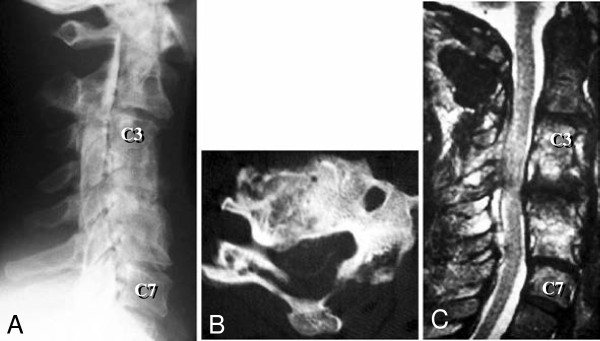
Three images obtained upon admission to our hospital (4.5 months after the patient’s third surgery). (A) A lateral view myelogram showing anterior compression of the dural tube at the C4-C5 level. (B) An axial computed tomographic myelogram showing that the spinal cord is compressed from the anterior and posterior directions at the C4-C5 level. (C) A T2-weighted midsagittal magnetic resonance image showing spinal canal stenosis at the C4-C5 level due to pseudoarthrosis of the C4-C5 fusion.
